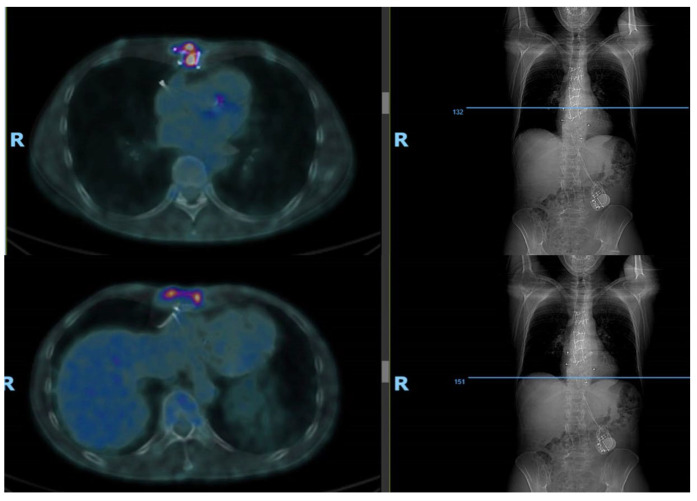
Osteomyelitis diagnosis—CT PET images documenting sternal osteomyelitis at various sections of sternum (proximally and distally).
